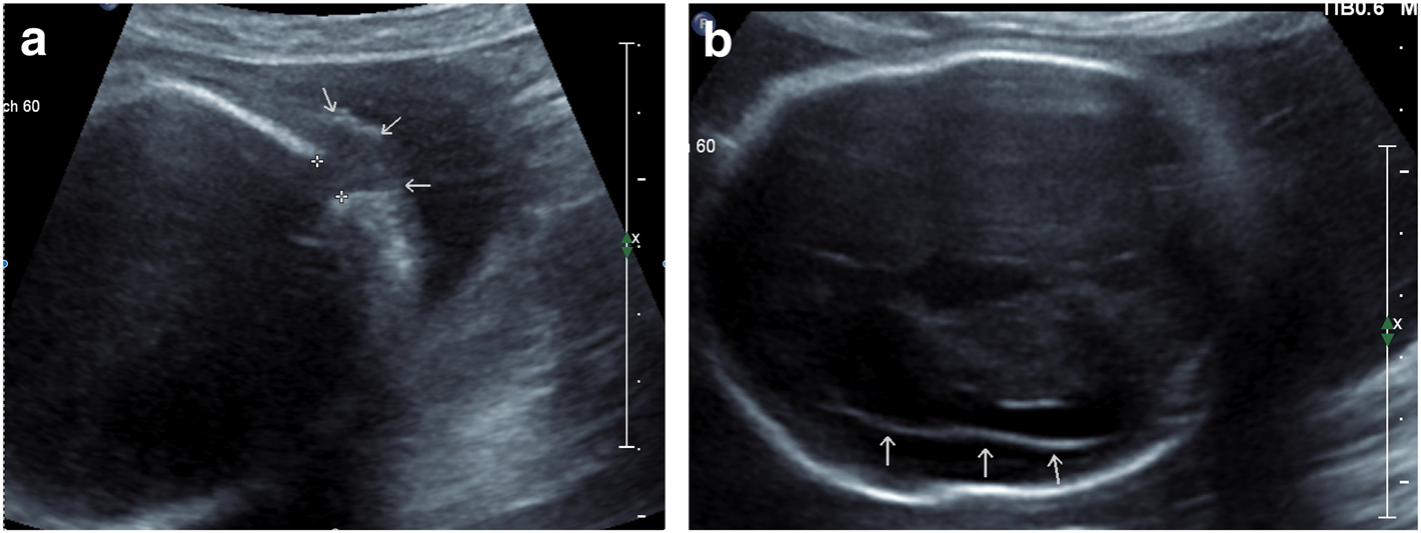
Prenatal ultrasound at 31 weeks of gestational age of a patient with Walker-Warburg syndrome (patient 25). a shows an occipital encephalocele (arrows) and b reveals absent gyration due to lissencephaly (arrows)
